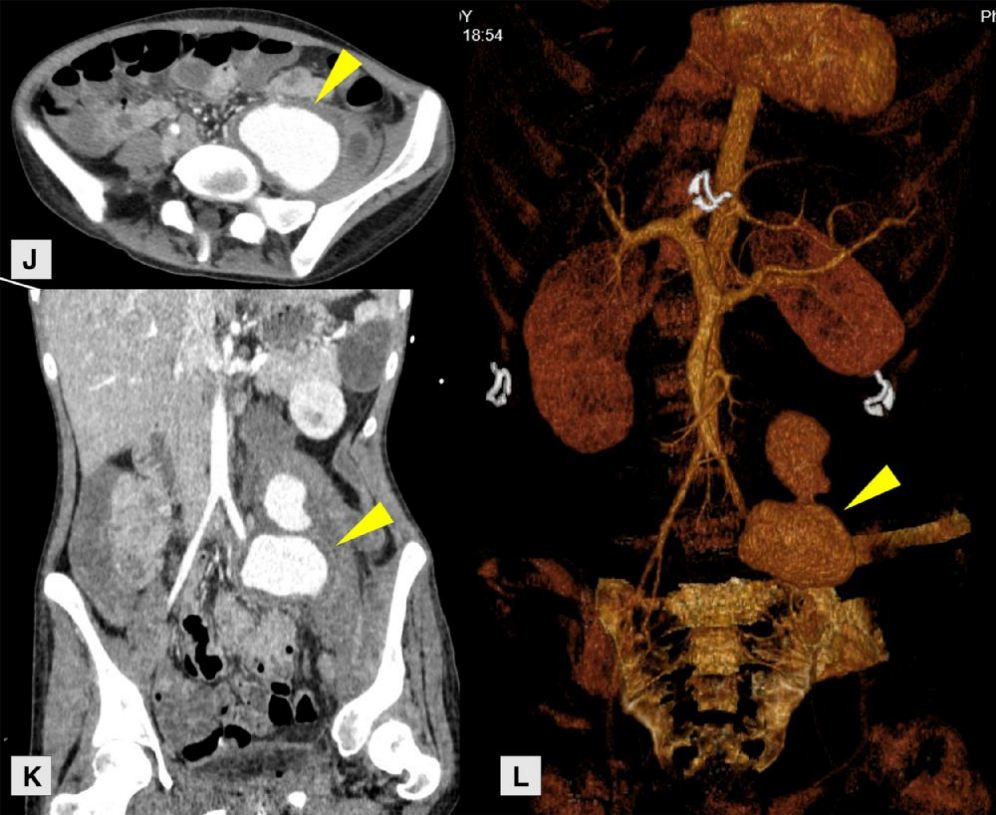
Computed tomographic angiography of the abdomen and pelvis revealing left internal iliac pseudoaneurysm rupture and haemorrhage into left psoas muscle (yellow arrowhead): (*J*) axial view; (*K*) coronal view; (*L*) 3D reconstruction of the left internal iliac pseudoaneurysm.
